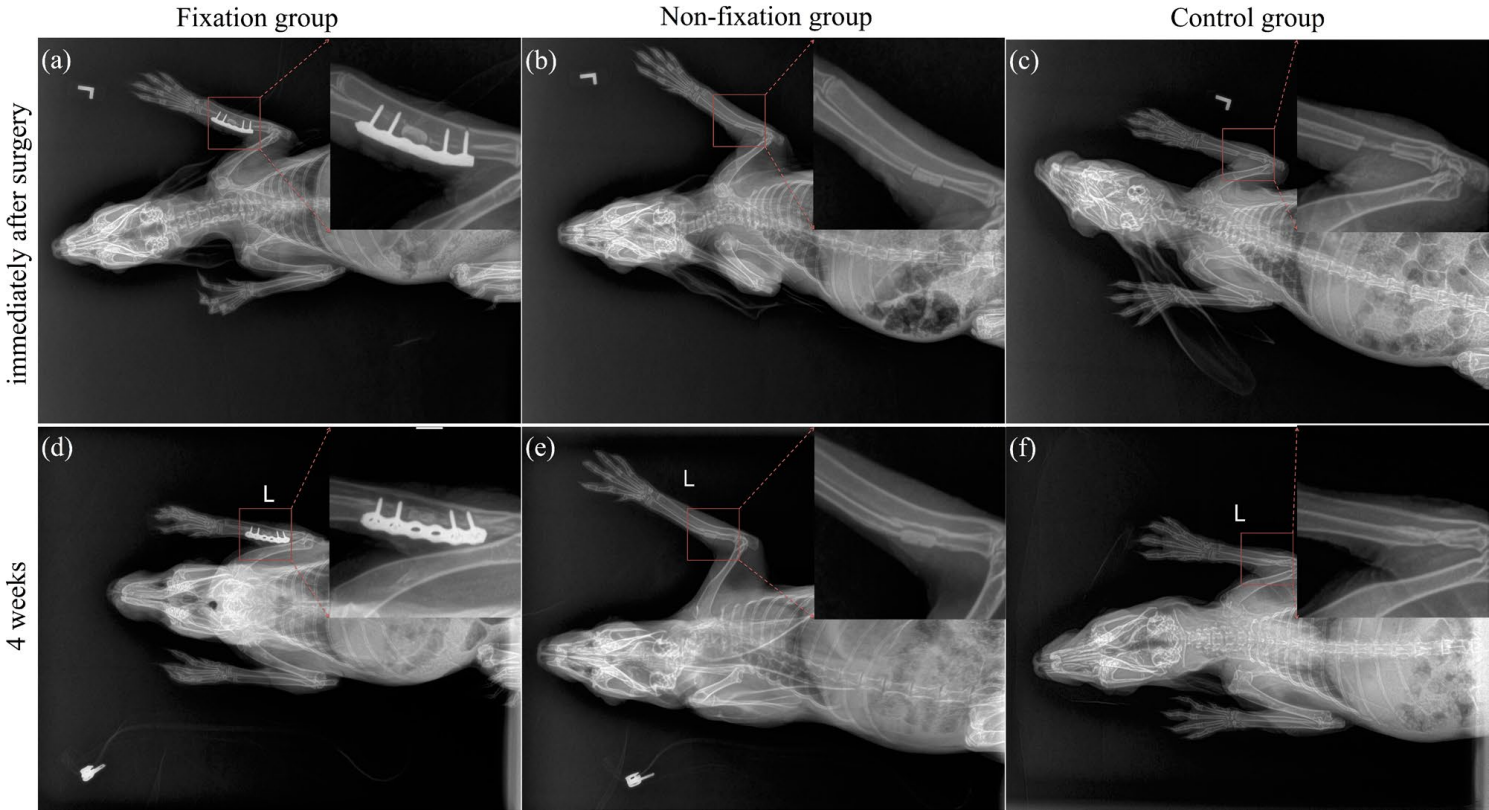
Postoperative X-ray images. (a–c) X-ray images shown that segmental left radius shaft defects were respectively fixed with PMMA and plates, PMMA only, or nothing, immediately after surgery. (d–f) There was no sign of plates loosening, or shift of PMMA, or immigration of fracture ends, 4 weeks postoperatively.
